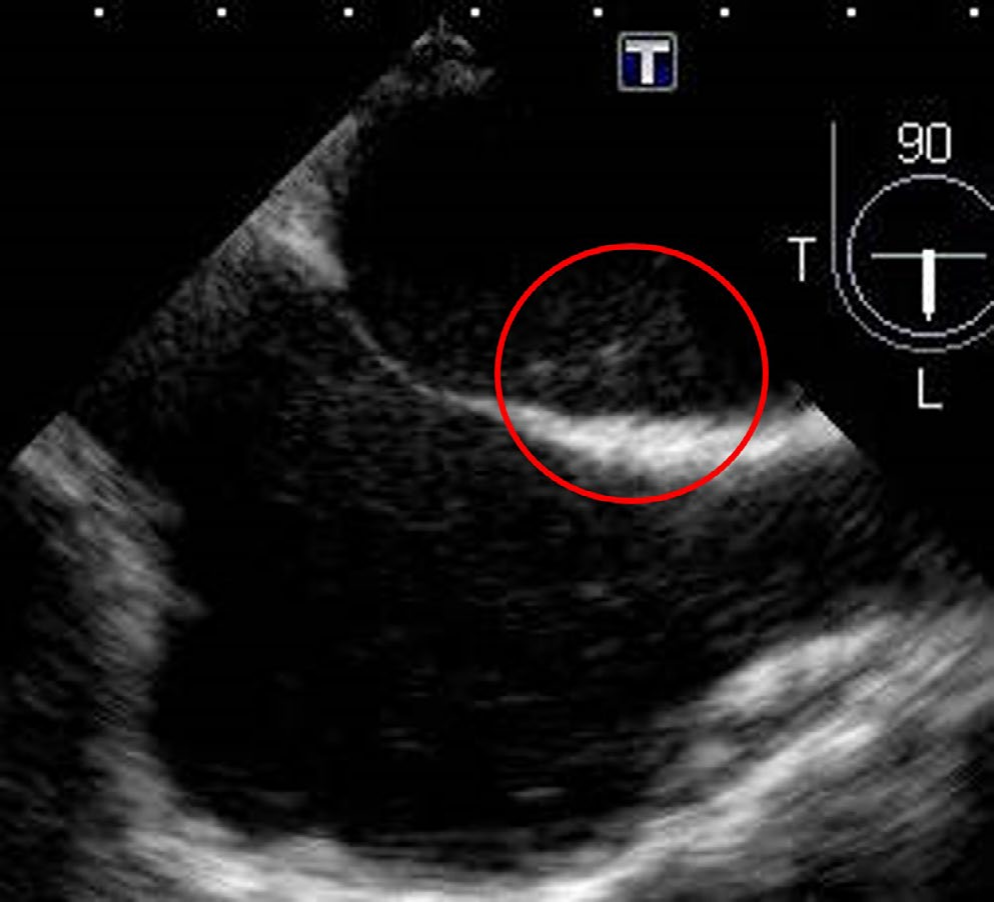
On transesophageal echocardiography, bubble contrast method showed time lag of the bubble about one or two breaths between the right and left atria
